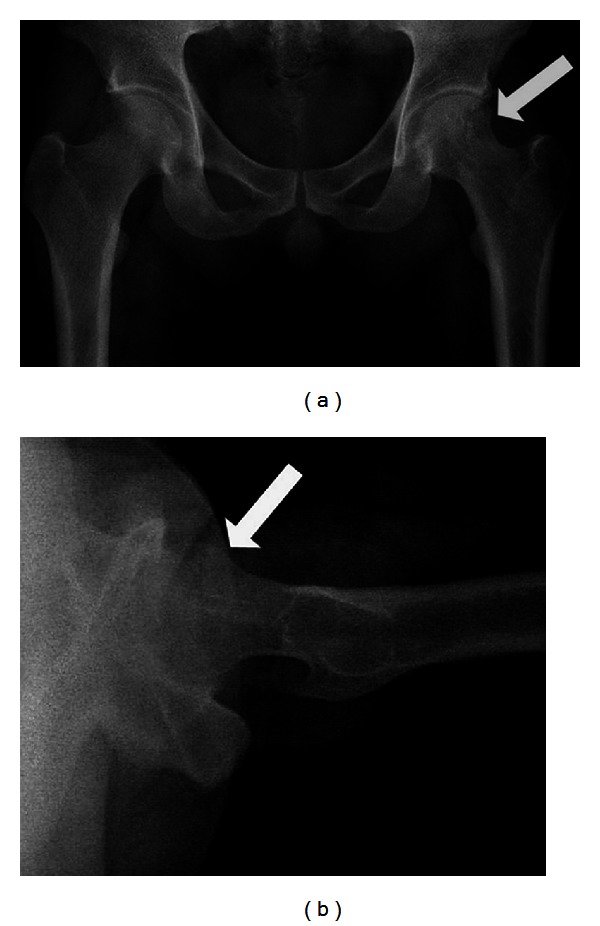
Radiograph taken 14 years after the initial surgery, showing the left neck of femur without the screws, and new trabeculae has formed over the left femoral neck with complete obliteration of the lesion.
